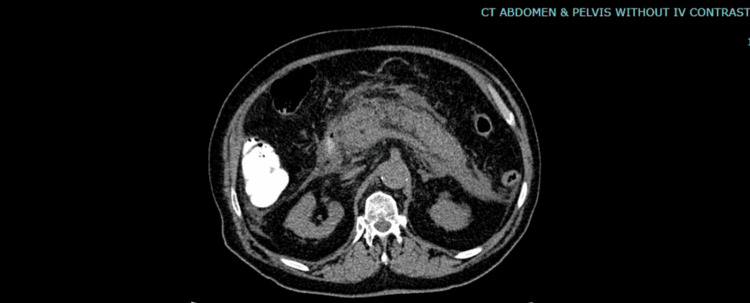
Computed tomography image of the abdomen and pelvis (without contrast) The image shows a swollen pancreas with no low attenuation areas with hazy and streaky induration surrounding it.
